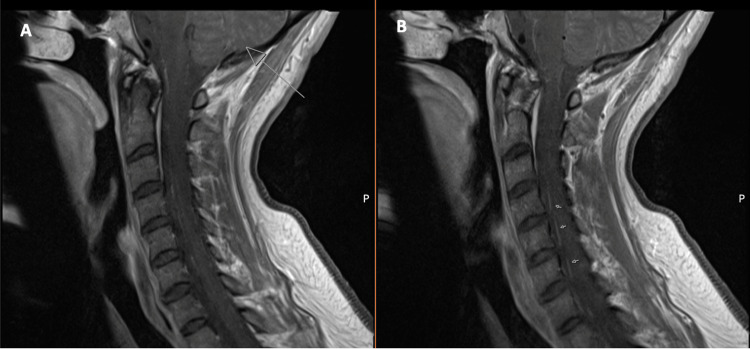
Sagittal postcontrast T1-weighted cervical spine MRI showing enhancement (arrows) of cerebellar folia (A) and multiple ventral nerve roots (B) MRI: magnetic resonance imaging
